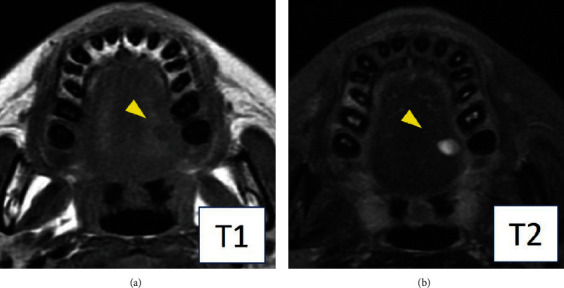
Magnetic resonance imaging findings. (a) T1-weighted axial image showing low signal intensity within the lesion (arrowhead). (b) T2-weighted axial image showing increased signal intensity within the lesion (arrowhead).
